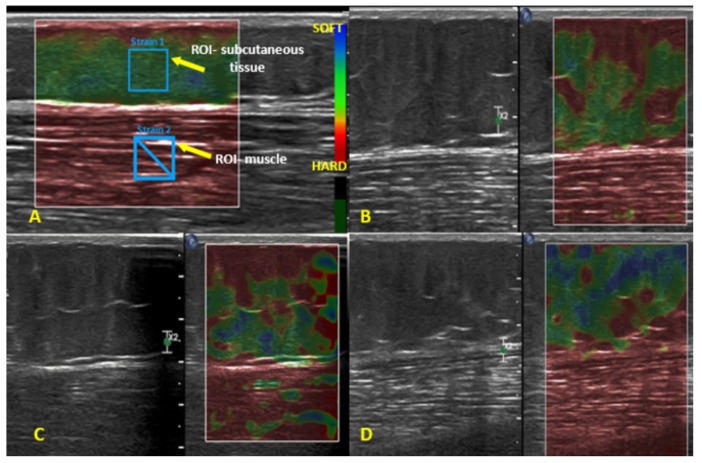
Ultrasound elastography of subcutaneous tissue (A). Ultrasound elastography of subcutaneous tissue: (B)—group 1; (C)—group 2; and (D)—group 3.
